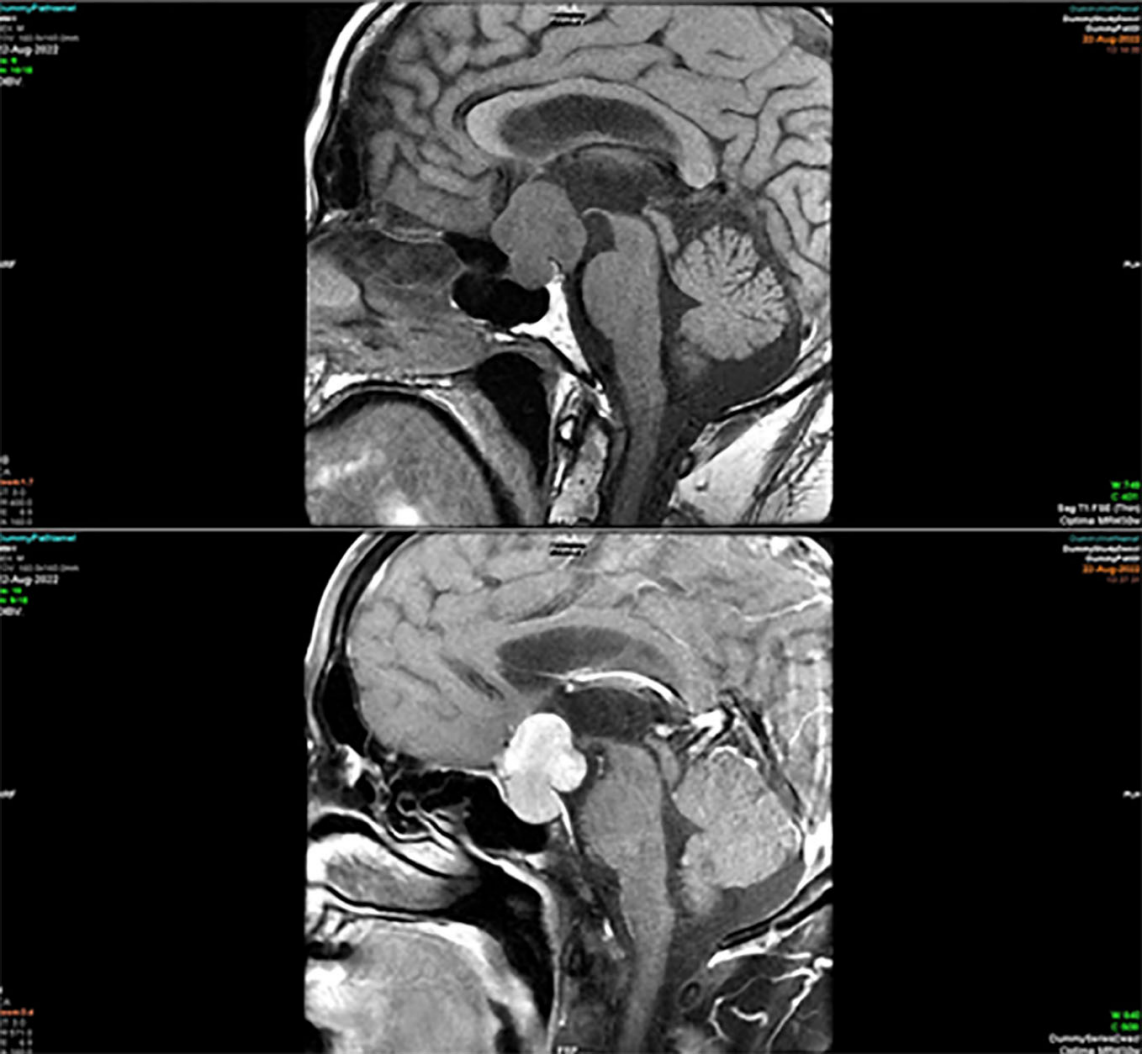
MRI T1 pre-contrast (top) and post-contrast (bottom) sagittal view of pituitary adenoma.
